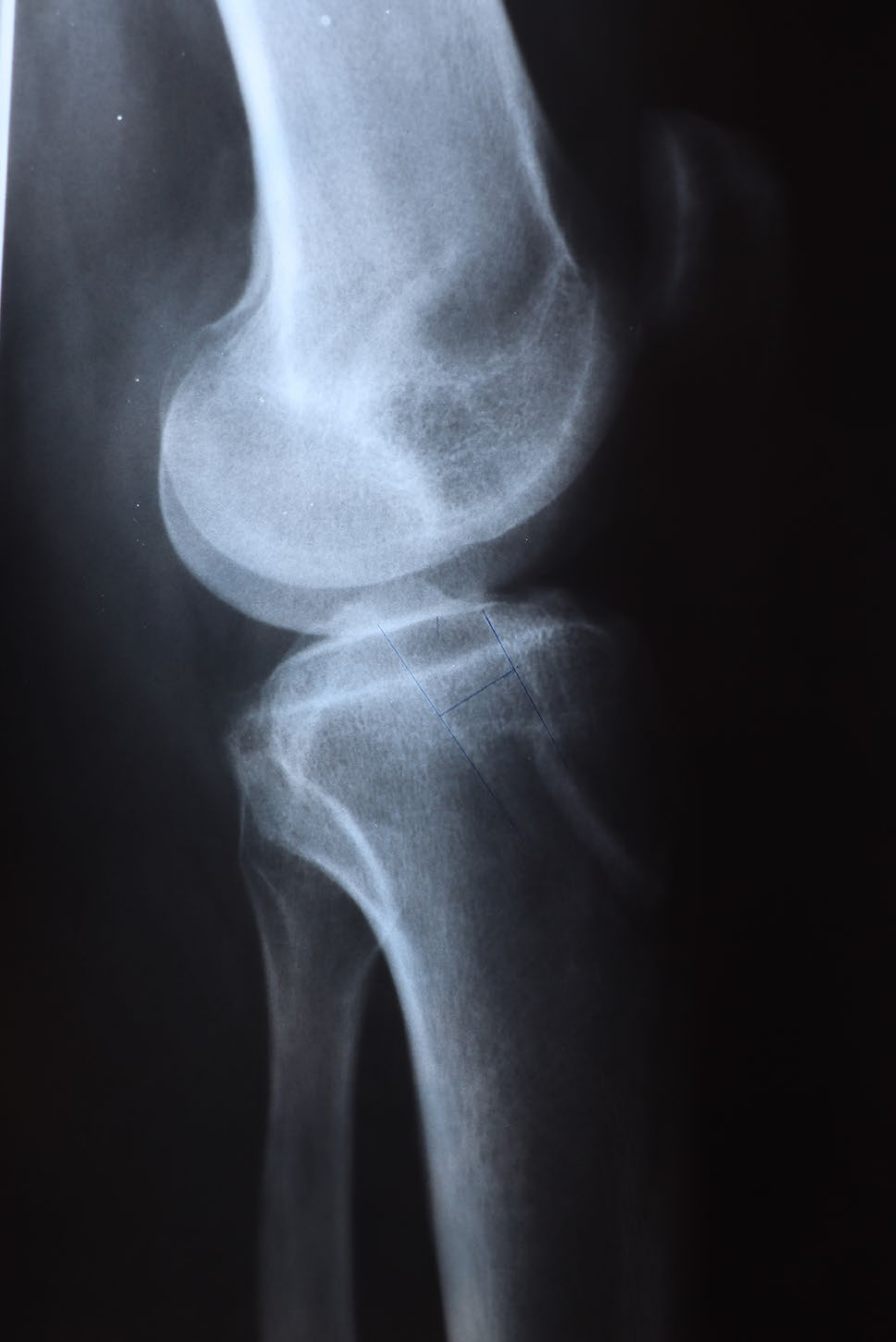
Tunnel widening on the lateral X-ray, using PT graft
